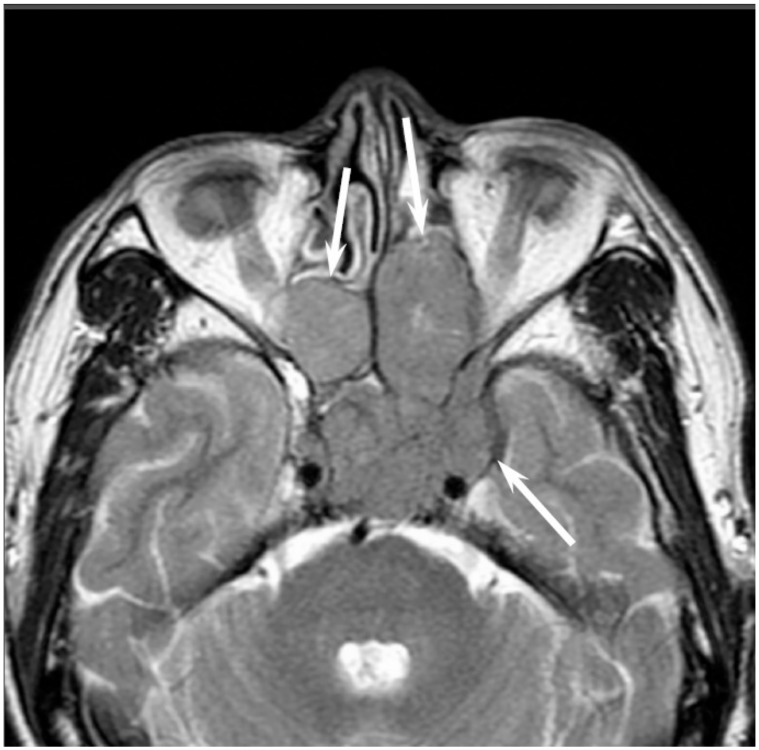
Extramedullary plasmacytoma of the bilateral nasal cavity. T2-weighted image showing a homogeneously isointense lesion (arrows).
